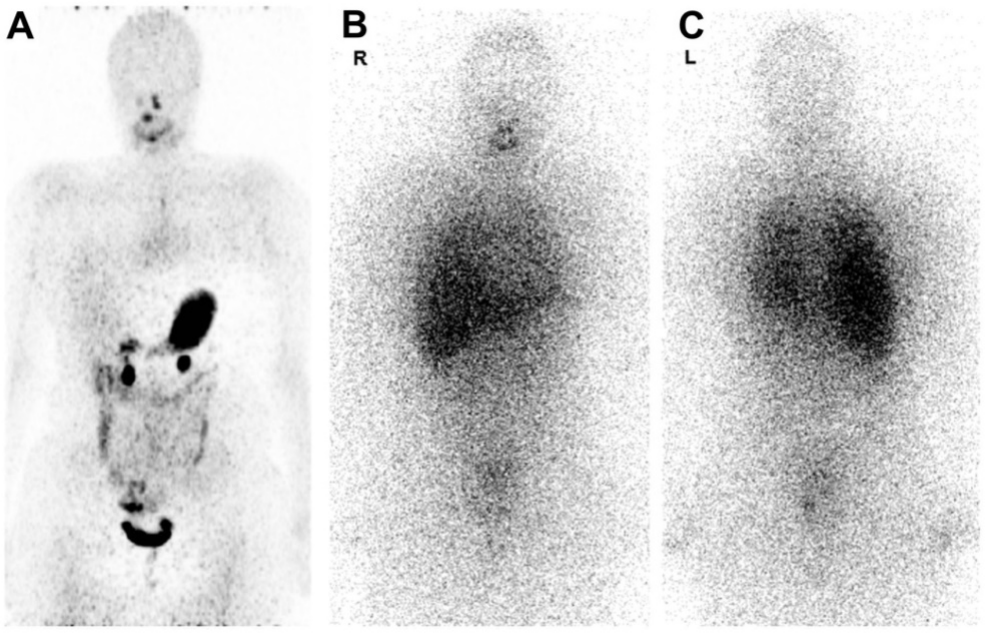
^124^I PET/CT image taken 1-4 days post-injection of 74 MBq (A). ^131^I WBS images taken 1 week post-injection of 5.5-7.4 GBq (anterior (B), posterior (C)). Adapted with permission from 141, copyright 2016, SNMMI.
